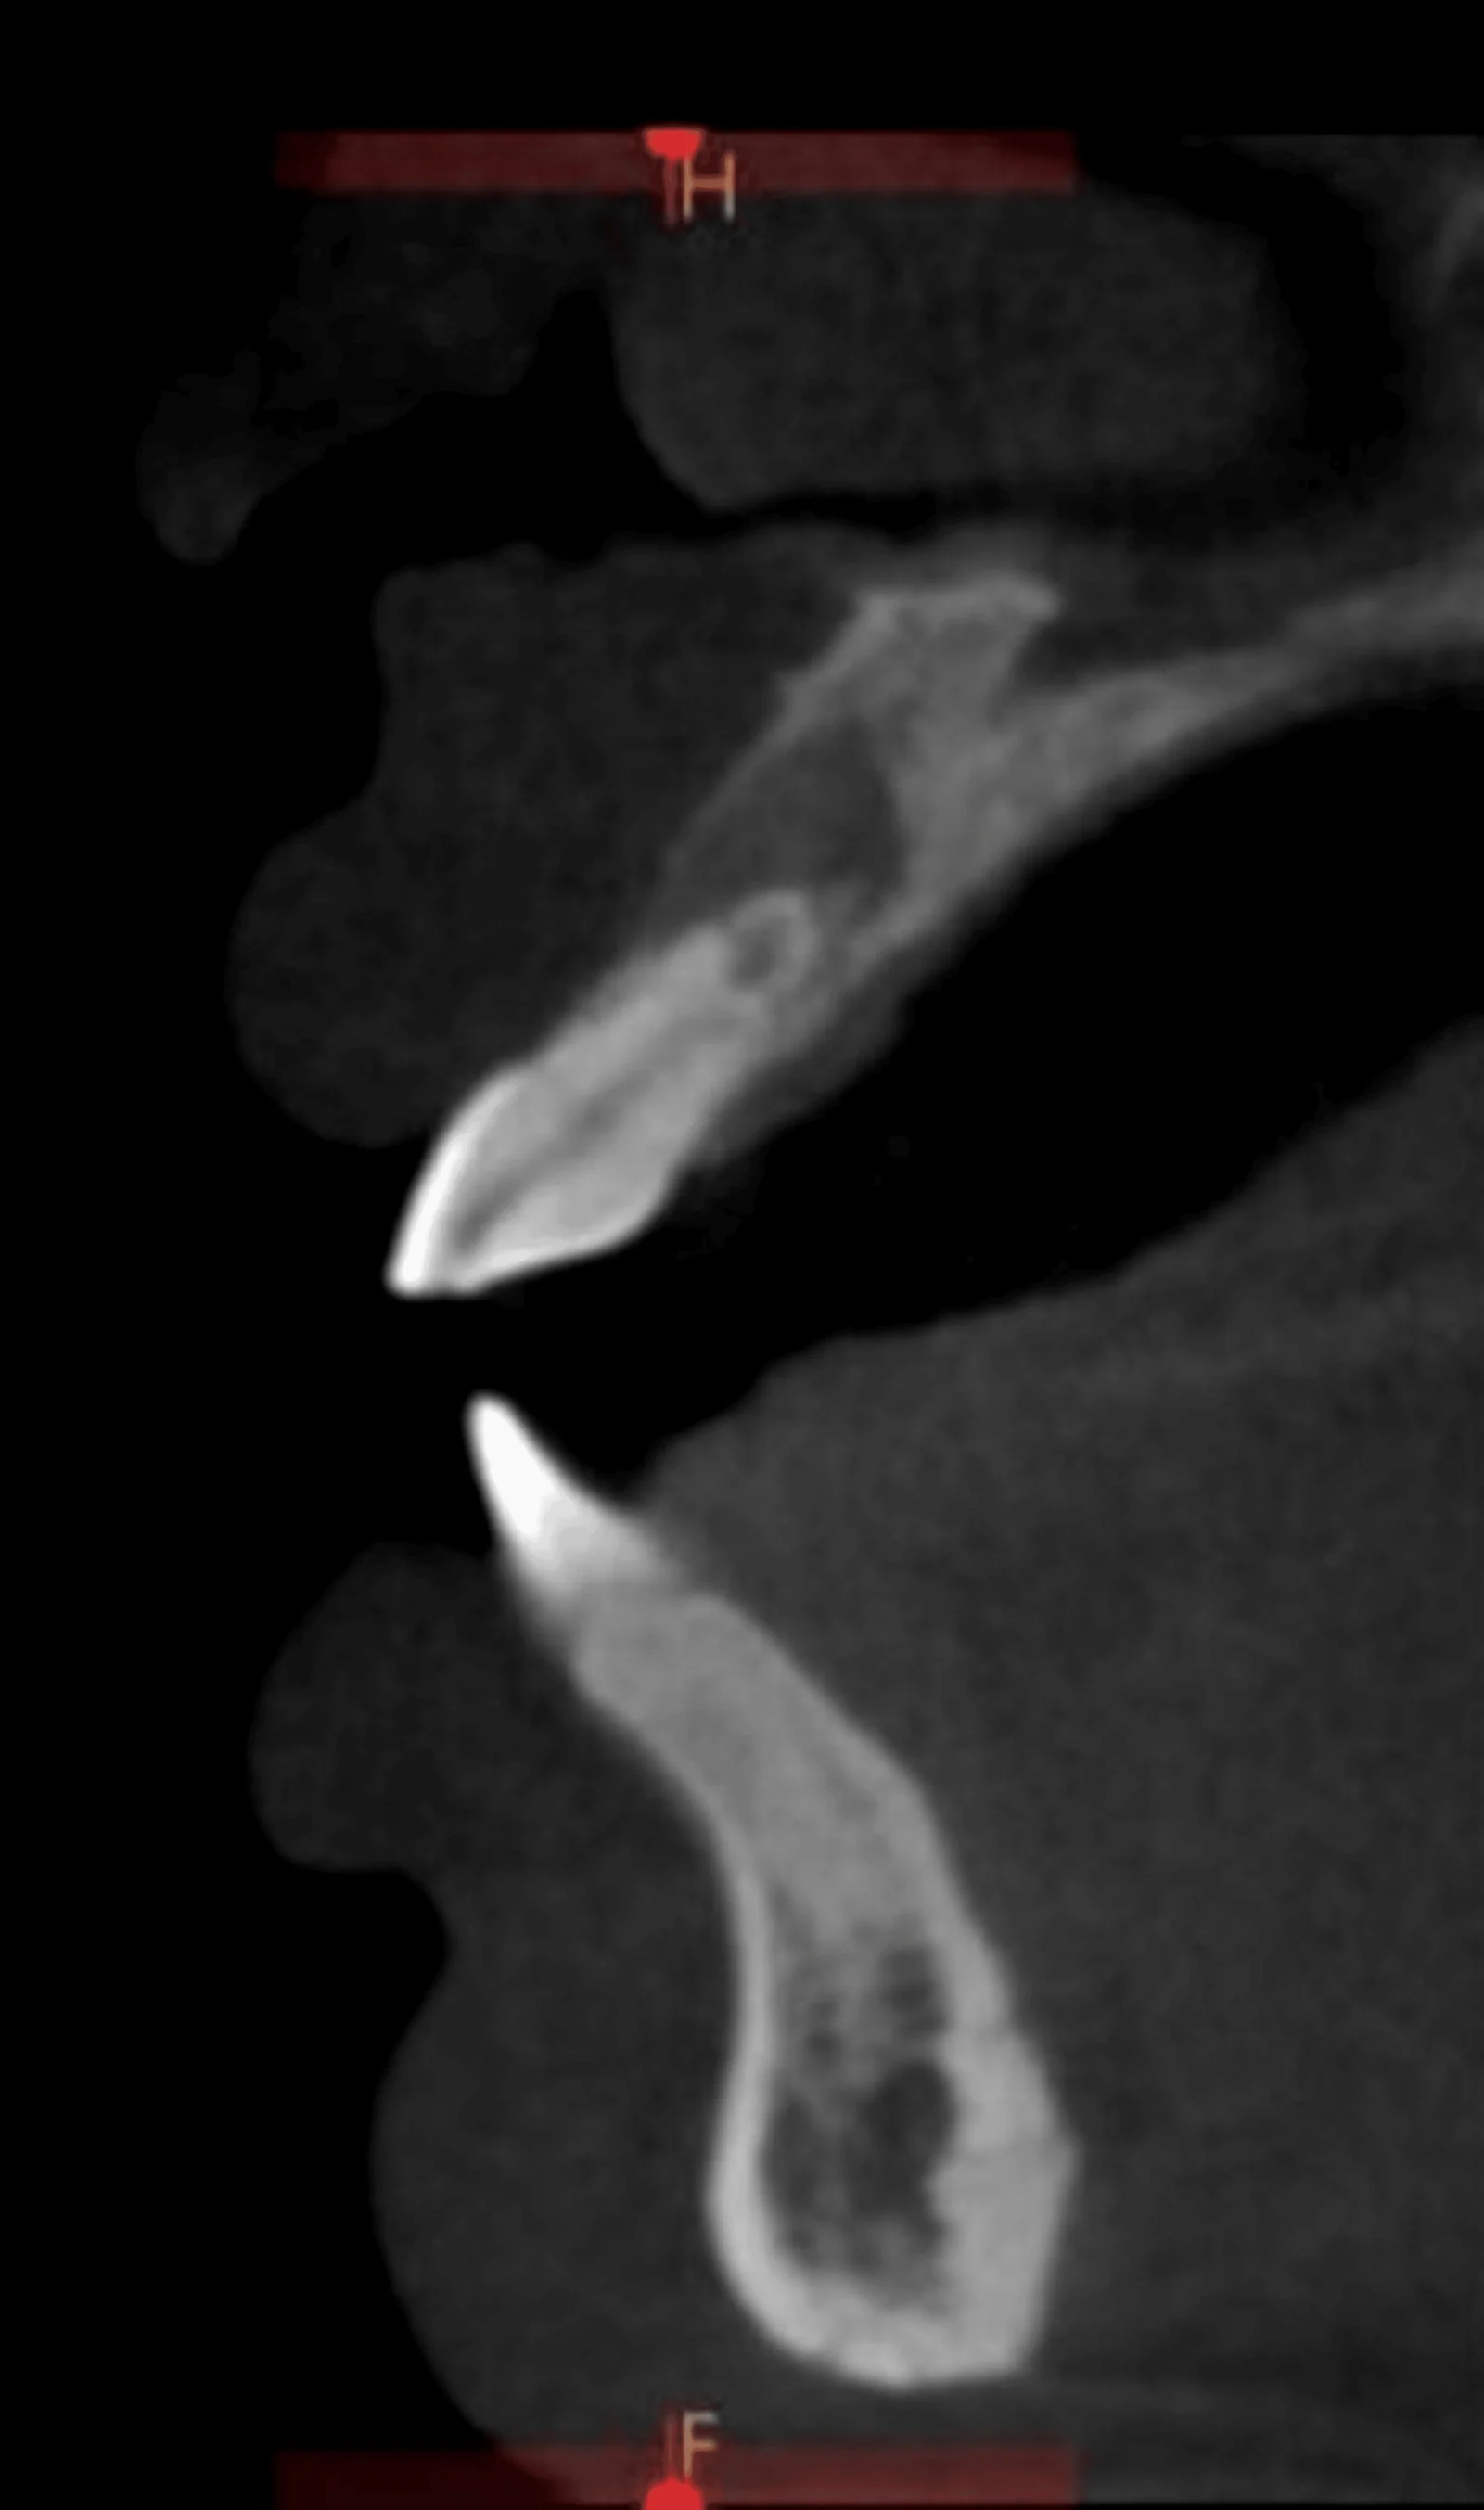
A periapical lesion at the apex of a non-endodontically treated tooth
